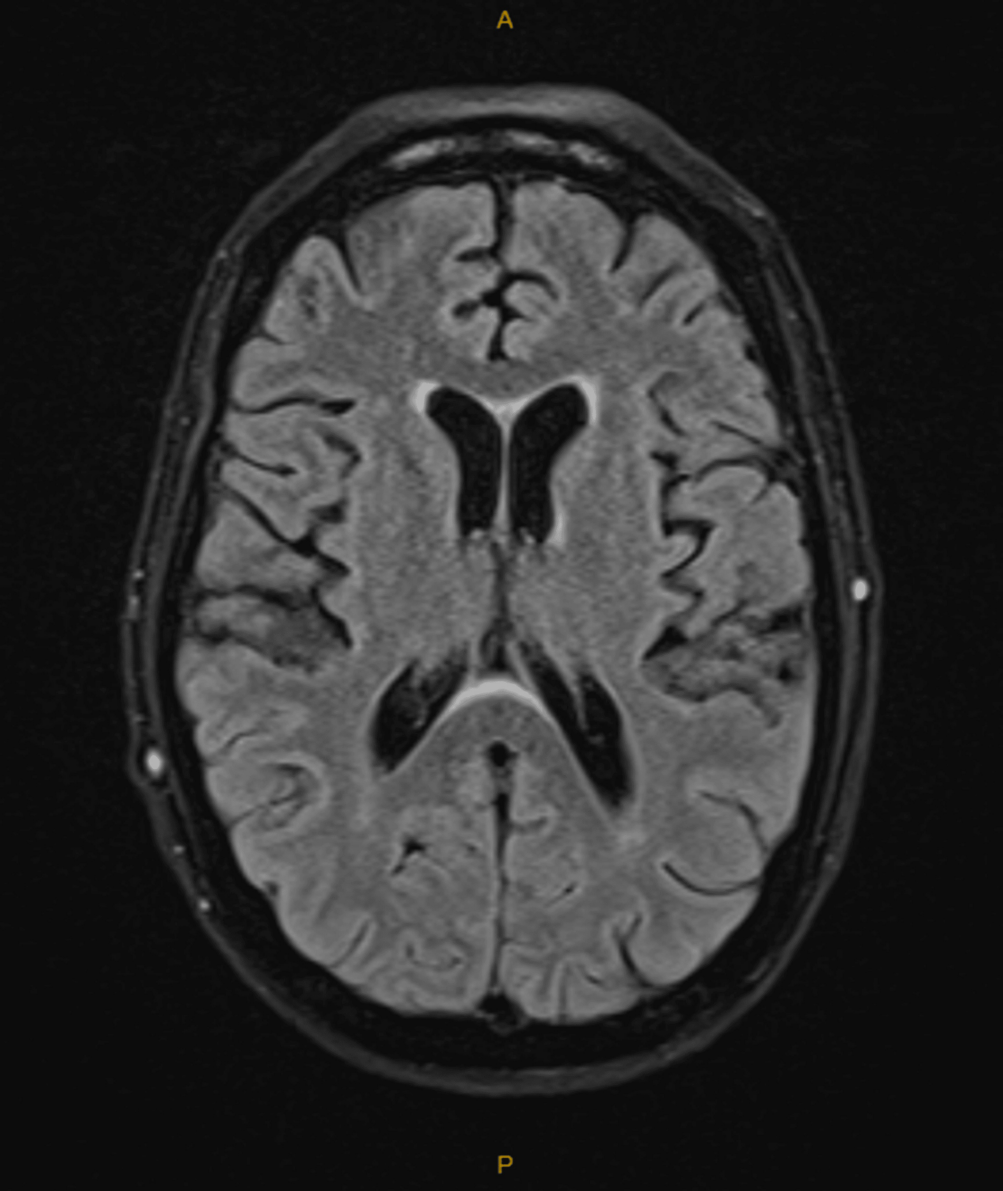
MRI Brain T2 FLAIR With Contrast Day 2 - Normal Study MRI: magnetic resonance imaging, T2 FLAIR: T2-weighted fluid-attenuated inversion recovery
